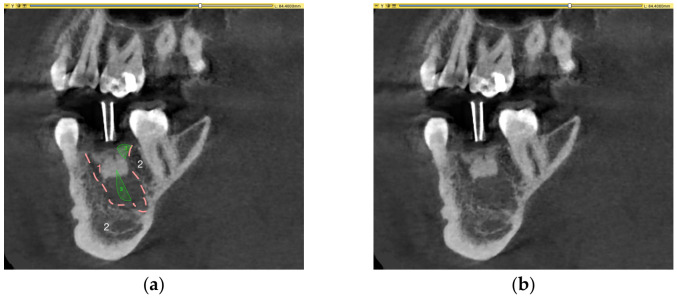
(a) CBCT with marked alveolus augmented with BO (1), surrounding jawbone (2) and defects that are part of the alveolus, contributing to an apparent volume gain (3); (b) part of the CBCT without marks.
